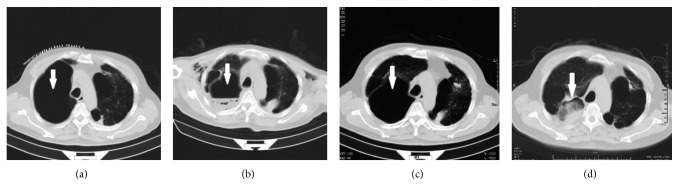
The patient 30 (case 32), a 59-year-old man, was complicated by pneumoconiosis, cor pulmonale, uarthritis, hypertension, and respiratory failure, had the GPB in the right lung (thick arrow) (a). The target GPB shrank to stable cavity in 30 days after the IBAP in March, 2013 (thick arrow) (b) until the second follow-up CT scan in April 2014 (thick arrow) (c). The right GPB closed completely observed at the third follow-up CT scan 3 years after IBAP in March 2016 (thick arrow) (d).
